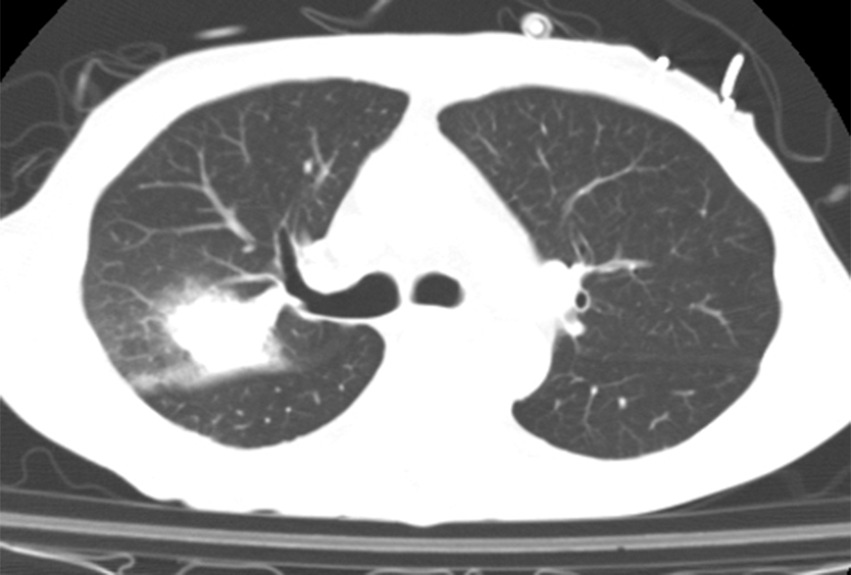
Axial chest CT image demonstrating a dense mass consolidation with surrounding ground-glass opacity in the right upper lobe.
